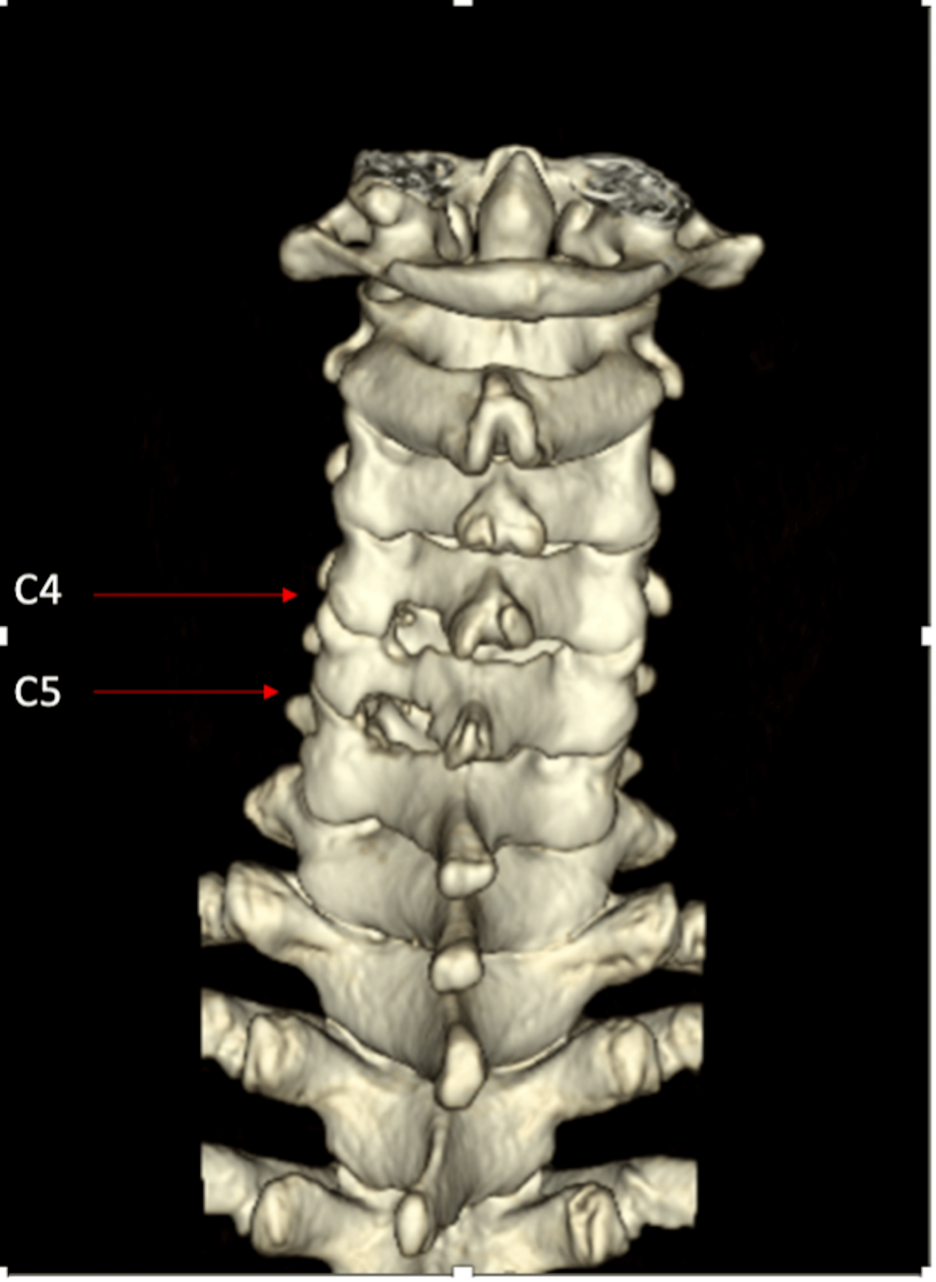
Postoperative 3D CT reconstruction image of the cervical spine, demonstrating the left C4/5 and C5/6 neural foramina are capacious after the left C4/5 and C5/6 laminoforaminotomy procedure (arrows).
